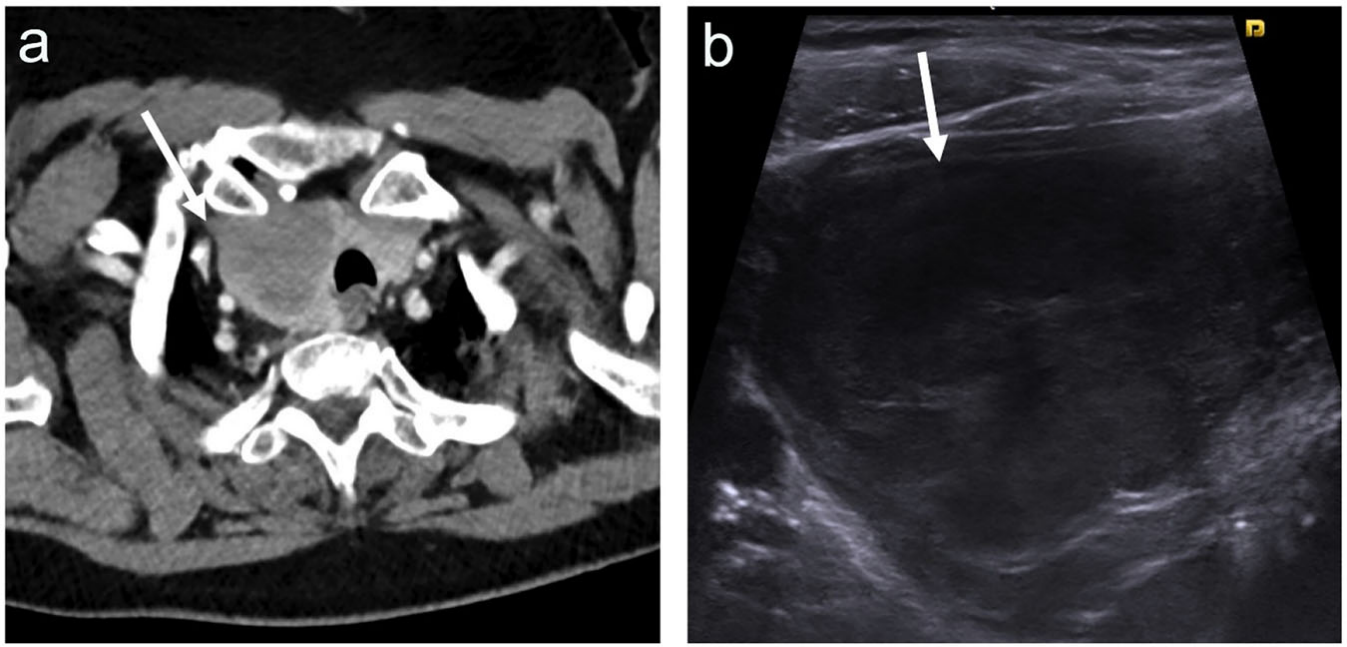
Incidental right thyroid lobe lesion with suspicion of extrathyroidal extension (ETE) on CT (arrow in (a)). On ultrasound RSS (b), the right thyroid lobe is replaced by a solid hypoechoic mass that bulges into the strap muscle (arrow), suggestive of malignancy. Core needle biopsy demonstrated diffuse large B-cell lymphoma
